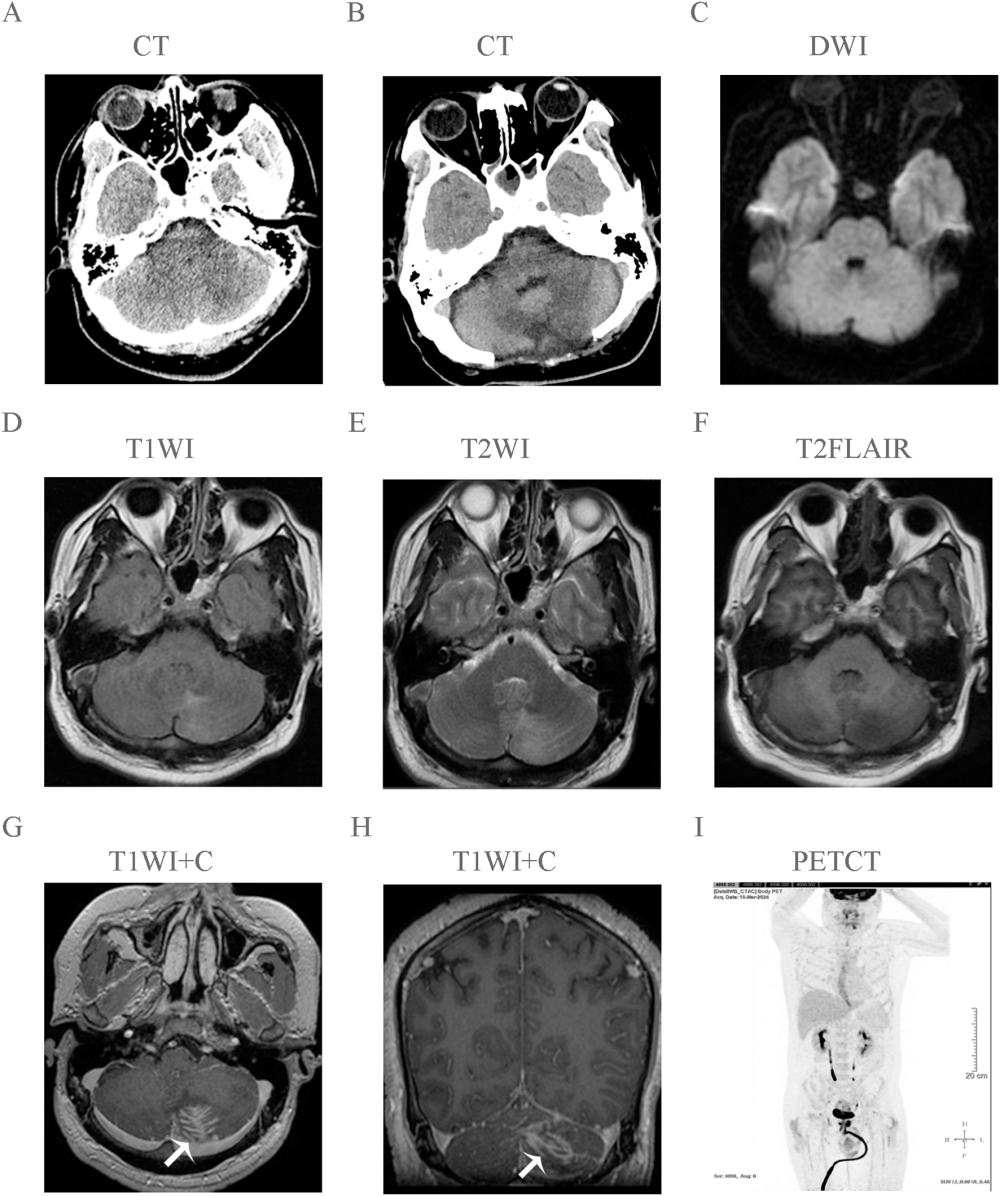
Multimodal neuroimaging of primary cerebellar ALK-positive anaplastic large cell lymphoma. (A) Preoperative non-contrast axial computed tomography (CT) reveals a patchy hypodense lesion in the left cerebellar hemisphere. (B) Postoperative non-contrast axial CT scan status after surgical resection. (C) Diffusion-weighted imaging (DWI) shows an isointense signal within the lesion, indicating no restricted diffusion. (D) Axial T1-weighted image (T1WI) demonstrates an irregular hypointense focus (arrow) in the left cerebellum. (E) Axial T2-weighted image (T2WI) reveals a confluent hyperintense area (arrow). (F) Axial T2-FLAIR image highlights perilesional hyperintensity (arrow) suggestive of vasogenic edema. (G, H) Axial and sagittal post-contrast T1-weighted images (T1WI+C) display delicate, linear “feather-like” enhancement patterns (arrows) at the lesion periphery. (I) Whole-body ^18^F-FDG PET-CT maximum intensity projection (MIP) image shows no abnormal extracranial metabolic activity, excluding systemic involvement.
